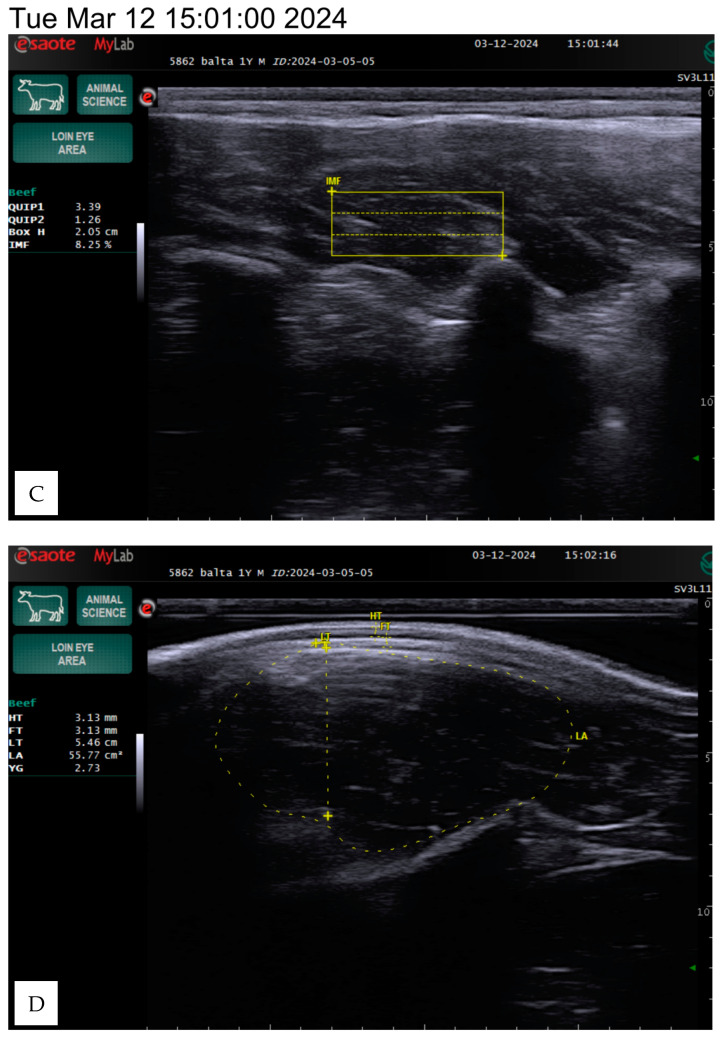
The example ultrasound images of the longissimus dorsi muscle of the Angus bulls, weights of 782 kg, IF 3.59% (A,B) and 485 kg, IF 8.19% (B,D). HT—hide thickness, FT—fat thickness (mm), LT—loin thickness (cm), LA—loin area (cm^2^), YG—yield grade, BH—box height (cm), IF—intramuscular fat (%).
